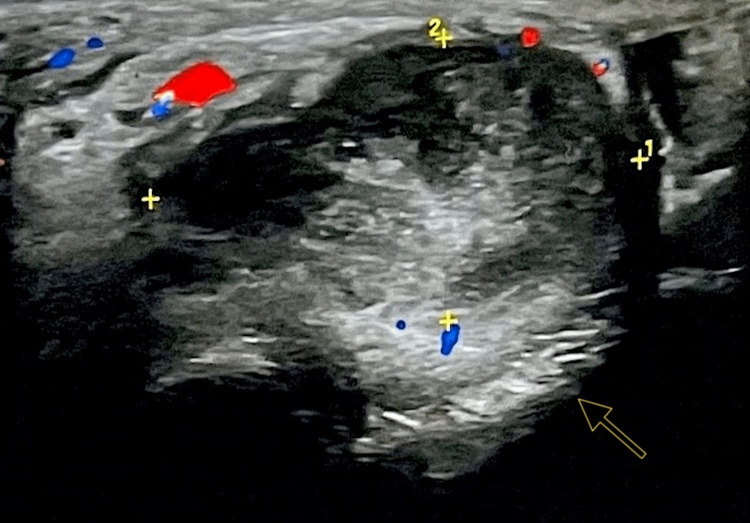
Ultrasonographic image of the left paratesticular lesion. Arrow indicating the paratesticular lesion, measuring (1) L 3,21 x (2) H 2,27. Doppler ultrasound measurements indicating blood supply of the lesion:
